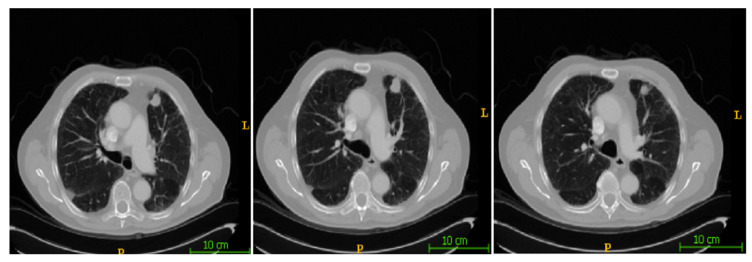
CT slices showing a solid lung nodule (Patient R_0108).
